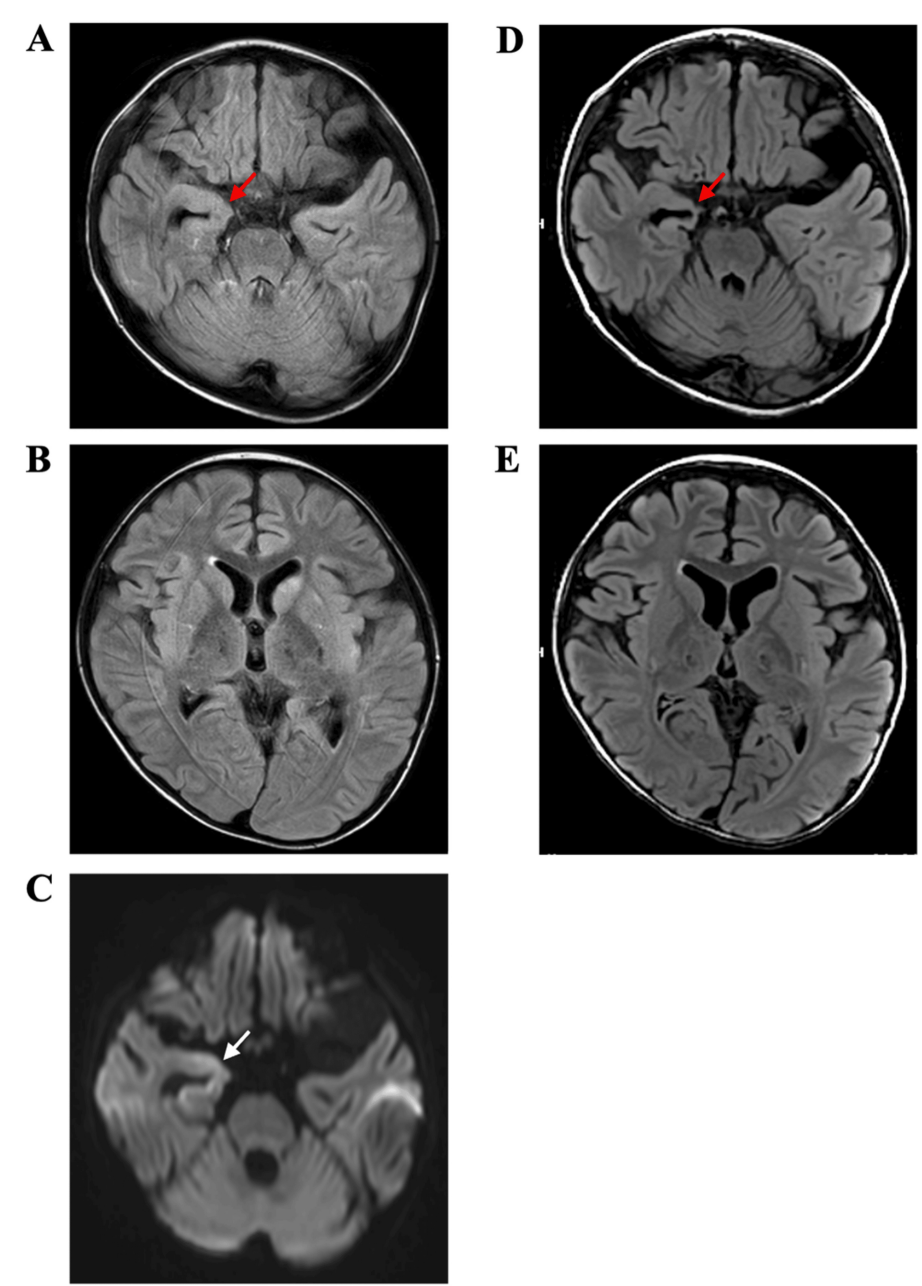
MRI of the patient's brain (A–C) Brain magnetic resonance images obtained in the acute phase after the onset of PALE 22 days after transplantation. (A, B) FLAIR images. (C) Diffusion-weighted image showing a high-signal area in the right hippocampus (white arrow). (D, E) FLAIR magnetic resonance images of the patient's brain acquired 17 months after the onset of PALE. Atrophy of the entire brain, mainly the right hippocampus (red arrow), is noted. FLAIR, fluid-attenuated inversion recovery; MRI, magnetic resonance imaging; PALE, post-transplant acute limbic encephalitis
